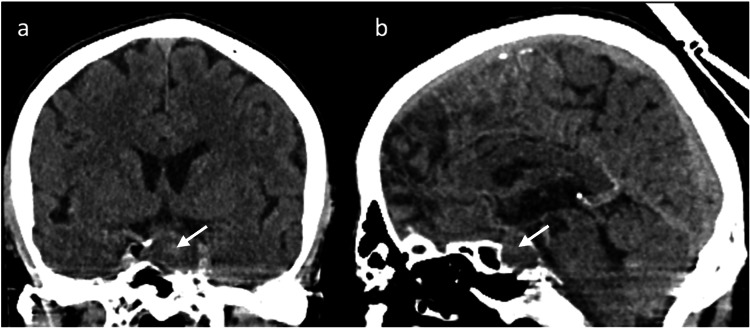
Follow-up CT five days after the initial CT shows coronal (a) and sagittal (b) images with progressive hypodensity of the sellar mass with some central hyperdensity (arrow). CT: computed tomography.
